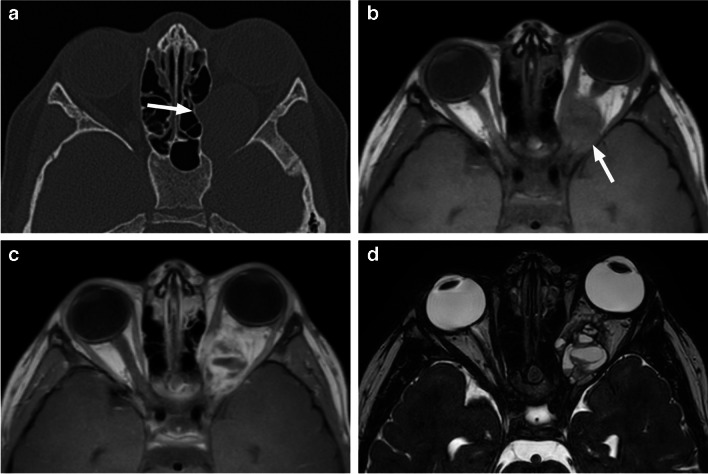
Axial CT image with bone algorithm and window (a) demonstrates mild expansion and smooth remodeling of the bony orbital walls on the left side with most prominent medial deviation of the lamina papyracea (arrow). There appears to be an intraconal mass lesion. Corresponding T1-weighted image (b) reveals a slightly heterogenous predominantly isointense intraconal mass (arrow), which exhibits partial patchy enhancement on the postcontrast image (c). High resolution 3D T2-weighted image (d) at the same level shows the characteristic fluid-fluid levels within the multicystic portion of this venolymphatic malformation (VLM)
